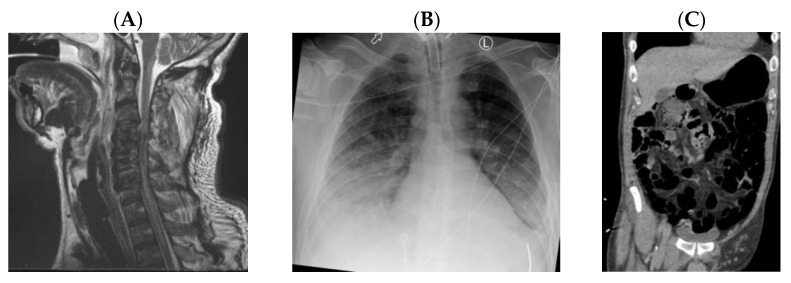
(A–C). (A) MRI cervical spine demonstrating a spinal cord contusion from C4 to C6. (B) Chest X-ray showing bilateral basilar consolidations suggestive of pneumonia and bilateral pleural effusions, which is worse on the right compared to the left. (C) CT abdomen and pelvis showing multiple loops of dilated bowel concerning for an ileus.
